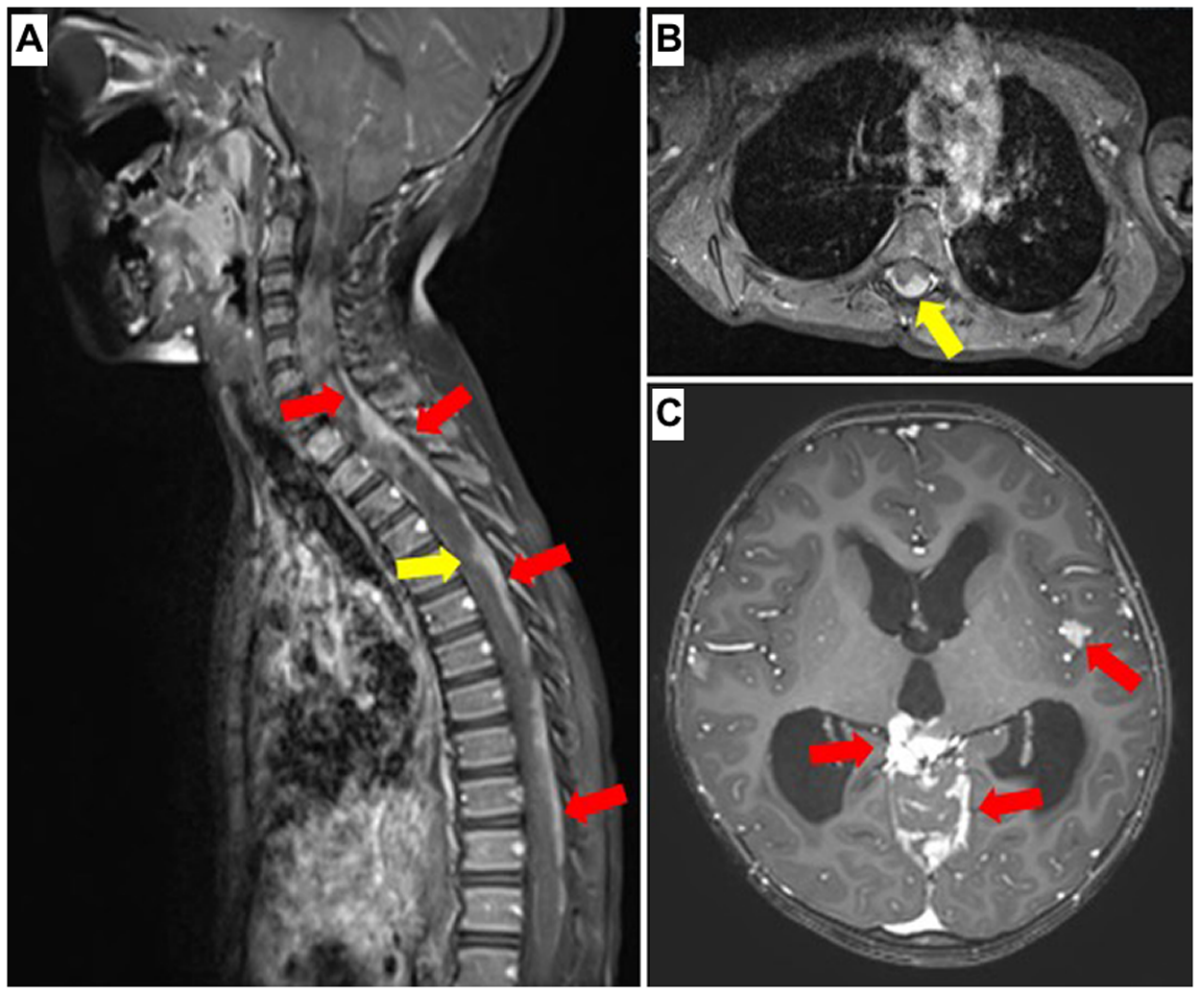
(A) Post-contrast T1-weighted MRI cervico-thoracic spine demonstrating extensive leptomeningeal enhancement along vertical axis of spinal dura. Red and yellow arrows depict leptomeningeal disease consistent with metastasis. (B) Yellow arrow correlates to same level of disease in the post-contrast T1-weighted axial image. (C) Representative image of T1-weighted post-contrast axial MRI with leptomeningeal enhancement, contrast-enhancement over areas in a‘sugar-coated’ manner and nodular disease, as highlighted by the red arrows.
